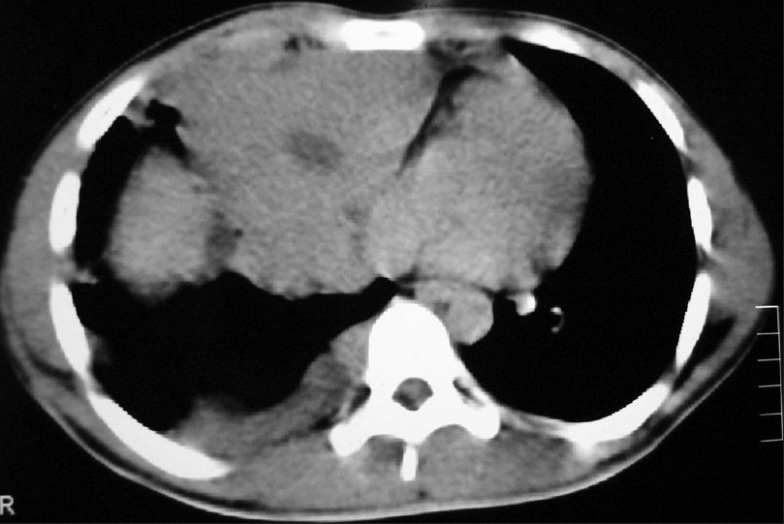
CT chest (2007 ) shows recurrence of anterior mediastinal mass extenting into right side of chest
